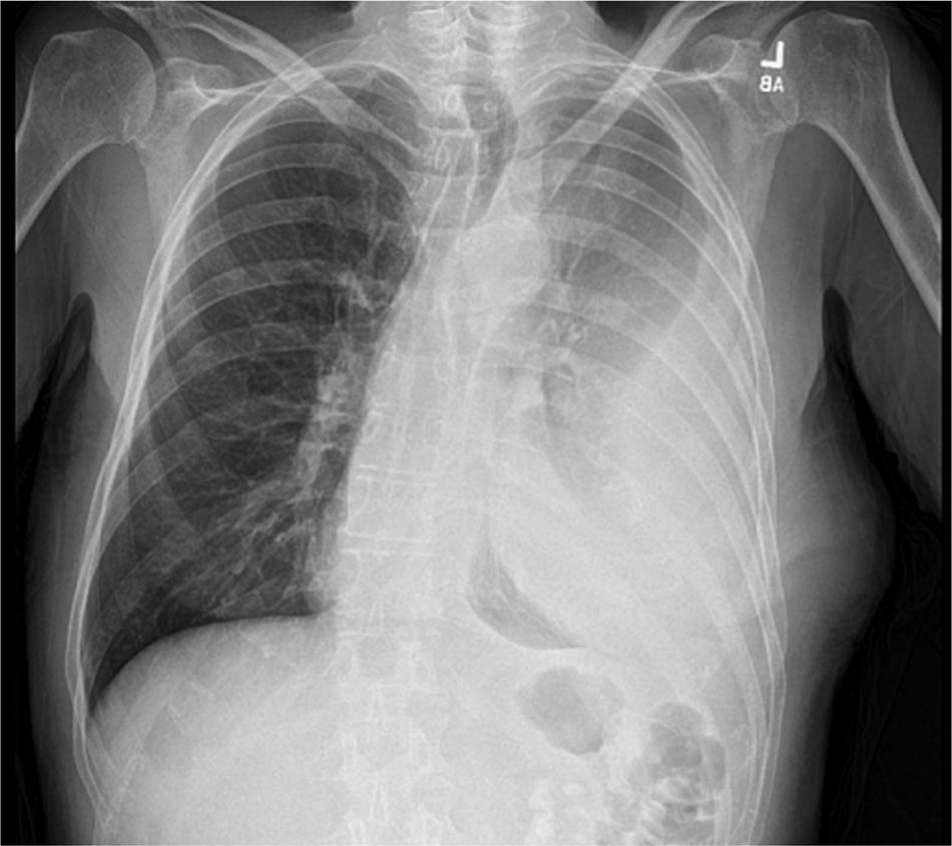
Initial chest radiograph showing a large loculated left sided pleural effusion.
